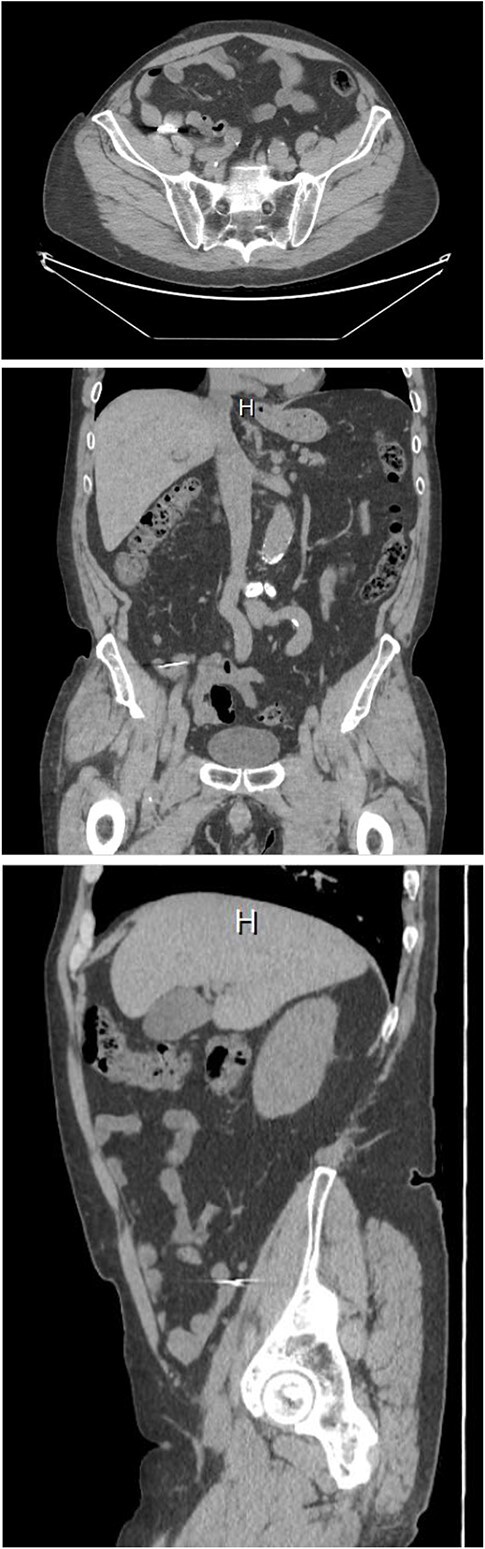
Computed tomography of the abdomen, axial, coronal, and sagittal views. The foreign body lies over the distal appendix. Detail is limited due to artifact.
